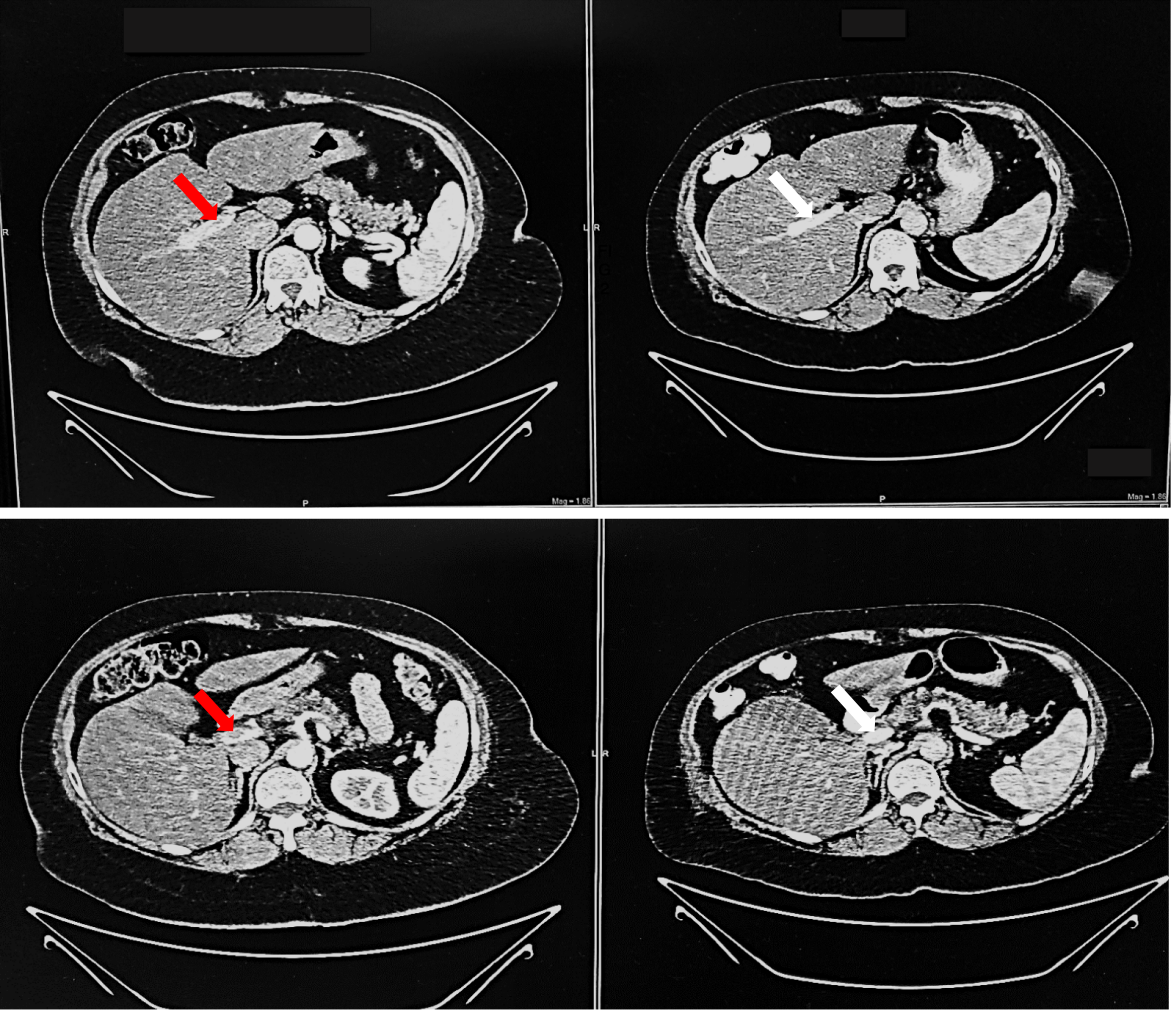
Cross-sectional views of abdominopelvic computed tomography scan with contrast comparing thrombosis of the portal vein (red arrows) before treatment and partial recanalization (white arrows) 3 days after beginning treatment
